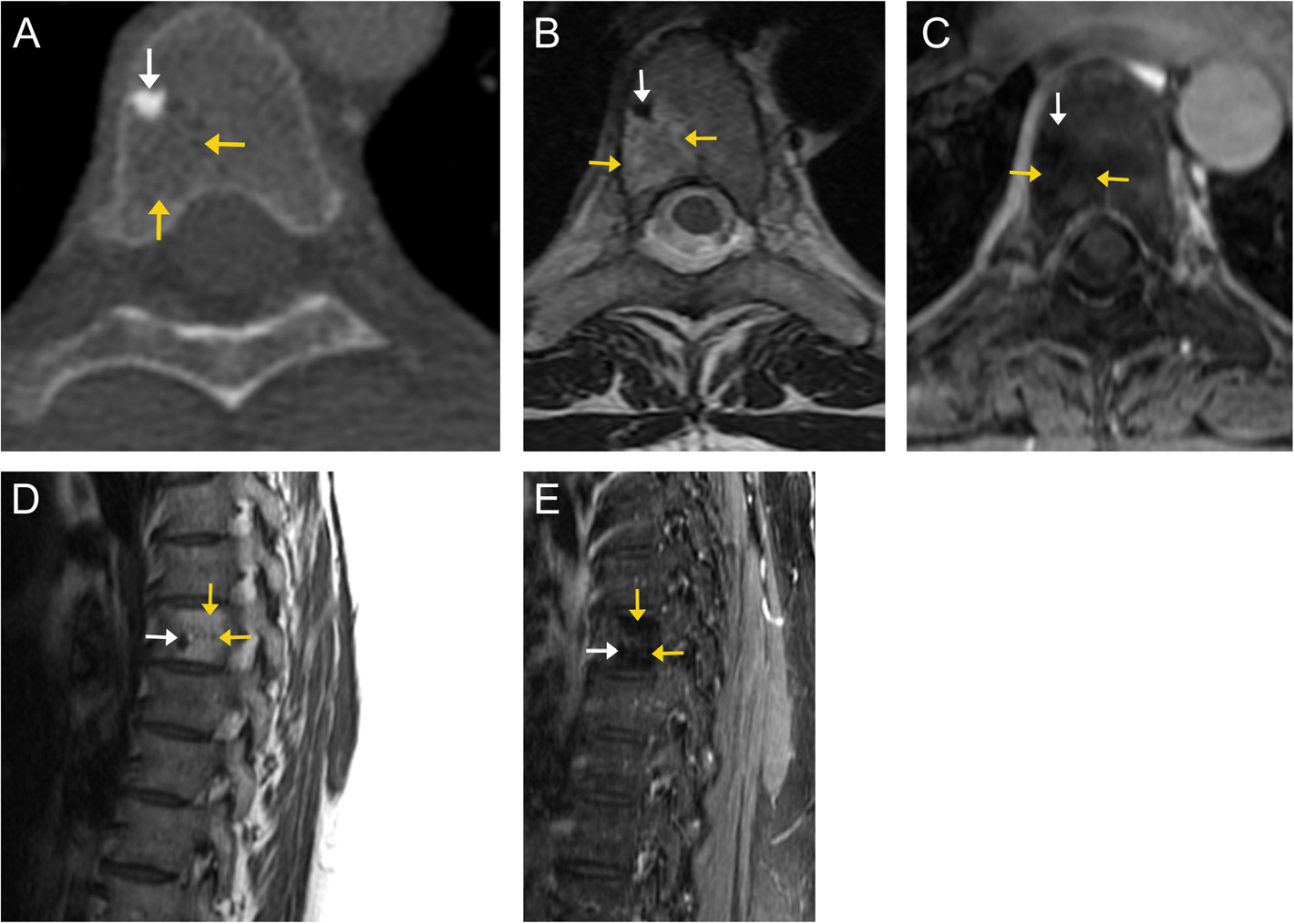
Enostosis with adjacent vertebral vascular malformation. Axial CT image of the spine (a) demonstrates the enostosis as a sclerotic bone lesion (white arrow). MRI shows low signal intensity like cortical bone; hypointensity on T1- (d, white arrow) and T2- (b, white arrow) weighted images, without enhancement post contrast (c, e). There is an adjacent ill-defined lesion with lucencies and intervening thickened trabeculae (a, orange arrows). This lesion demonstrates T1/T2 hyperintense signal (b, d, orange arrows), fat suppression (c, e, orange arrows) and no enhancement post contrast (c, e, orange arrow). This lesion is consistent with a vertebral venous vascular malformation (formerly known as a hemangioma)
